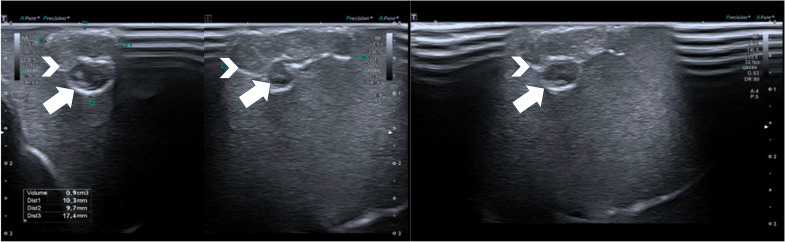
Presurgical intraoperative ultrasound. Right testicular intraparenchymal lesion (arrow), adjacent to the tunica albuginea at its anterior margin (arrow head), hypoechoic, polylobulated, circumscribed with peripheral echogenicity, with slight peripheral flow on color Doppler.
